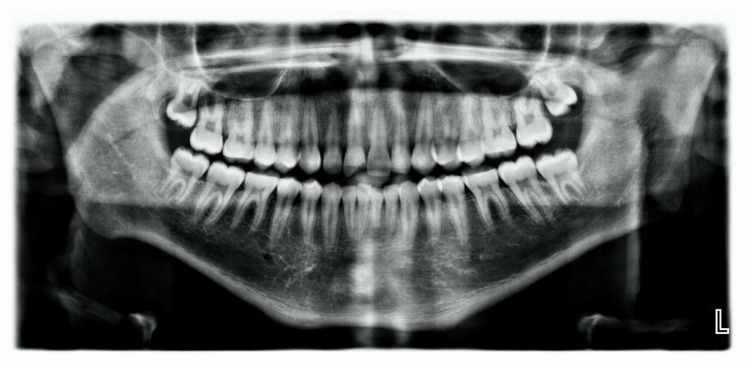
The ASD patient’s orthopantomogram shows a dentition free of caries and restorations, and the diagonally fractured incisal edge of tooth 11. No apical translucency or widened periodontal gap is visible. (©Witten/Herdecke University).
